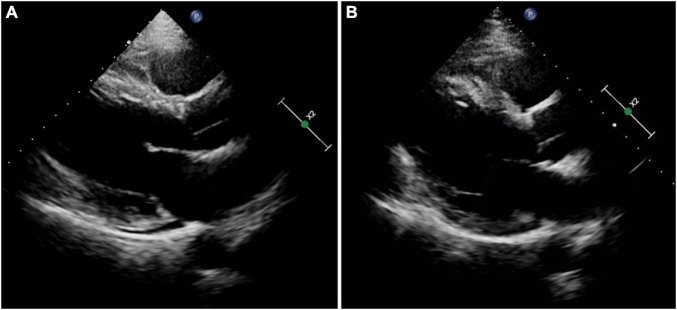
Results of transthoracic echocardiography. (A) On admission. (B) At the 3-month follow-up.
